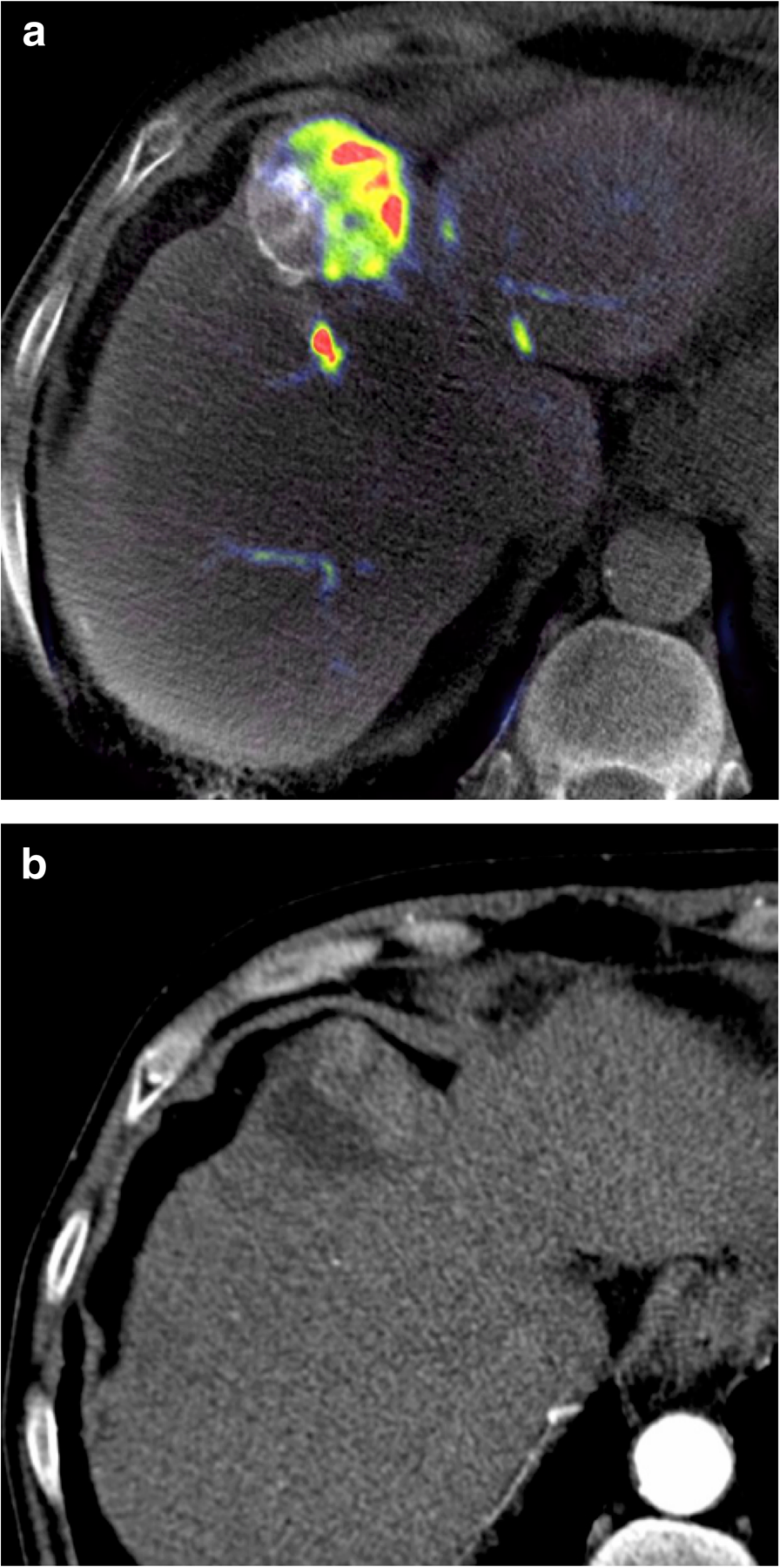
a C-arm CBCT PBVI performed immediately after TACE via the artery to segment VIII showing high density contrast in the treated portion of the lesion. There is persistent viable tumor supplied via segment IVa. b Follow-up arterial phase MDCT confirming persistent viable tumor supplied via segment IVa
